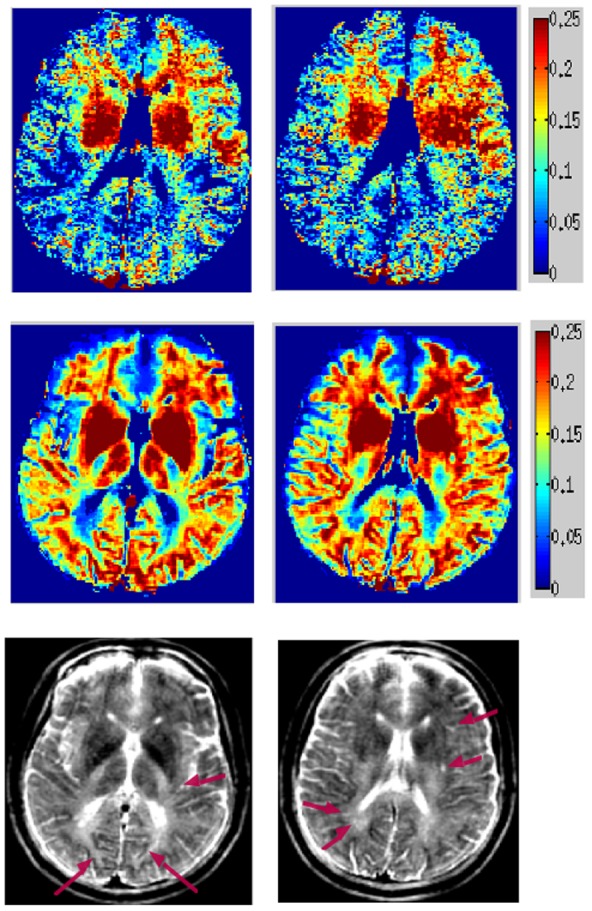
MWF maps of MS patient using conventional and spatially constrained method. MWF maps computed from *in vivo* MS patient scan. Top to bottom are MWF maps from conventional method, spatial constrained method and T2-weighted image at 60 ms. Arrows in T2 of point to small, focal lesions, externally confirmed on FLAIR. These lesions are well delineated on the spatial map but not on conventional map.
